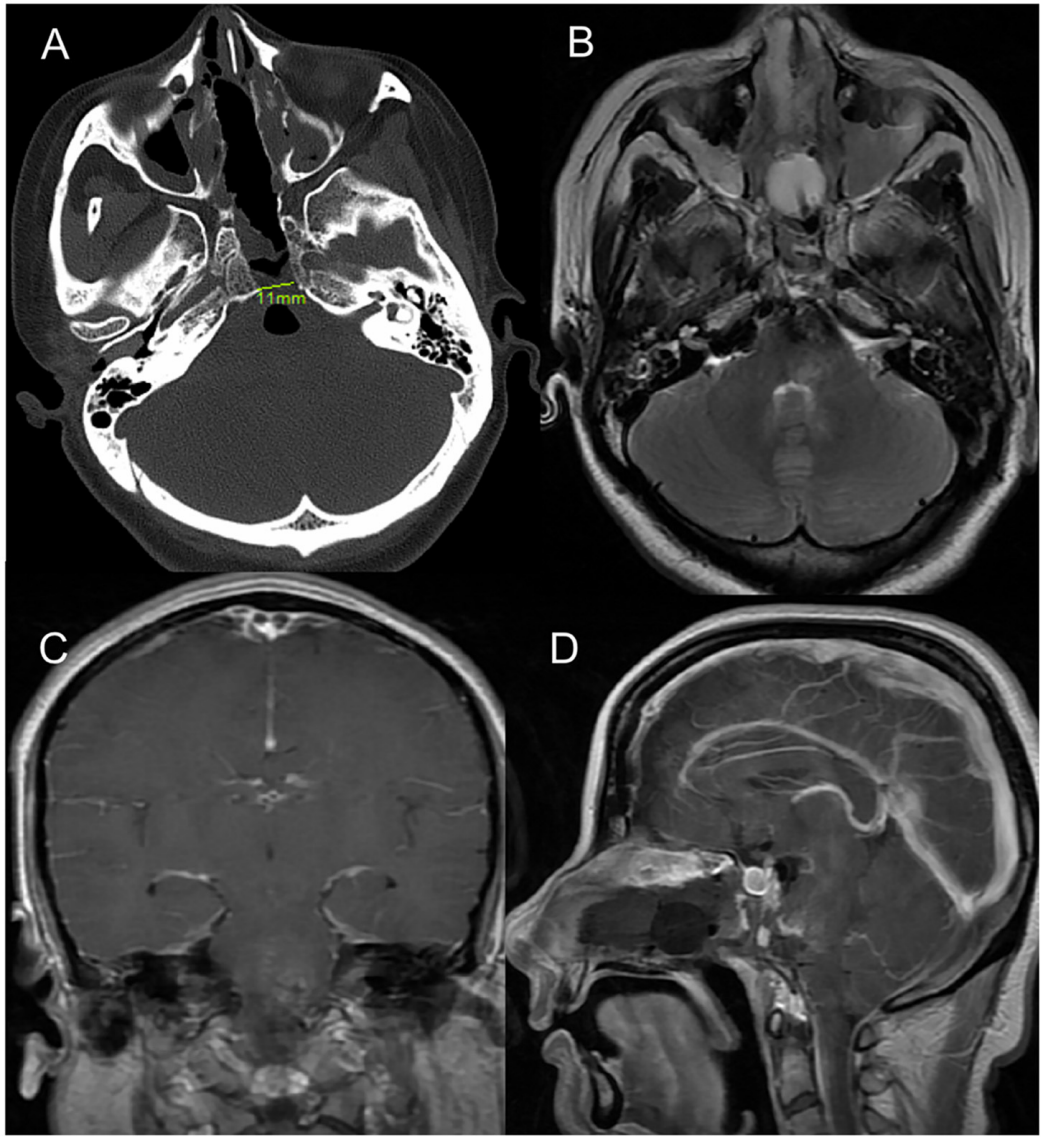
Postoperative imaging. (A) Post-operative axial CT image (bone window) indicating the drilled clival bone with a very limited opening of only 11 mm. (B–D) Axial T2-weighted, coronal and sagittal T1 postcontrast magnetic resonance image demonstrated no residual CM in ventral pons.
